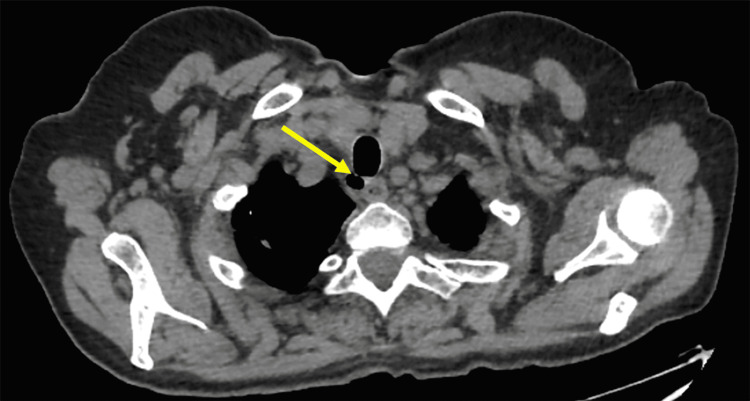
CT scan of the chest showing the tracheal diverticulum manifesting as a pouch on the right posterolateral wall.
